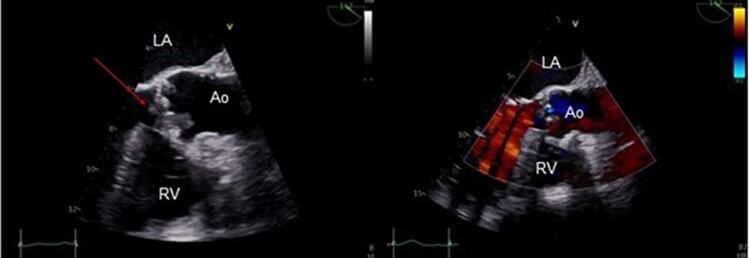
Transoesophageal echocardiography of Patient 2. The arrow indicates the aortic valve and the vegetations. Ao, aorta; LV, left ventricle; RV, right ventricle.
